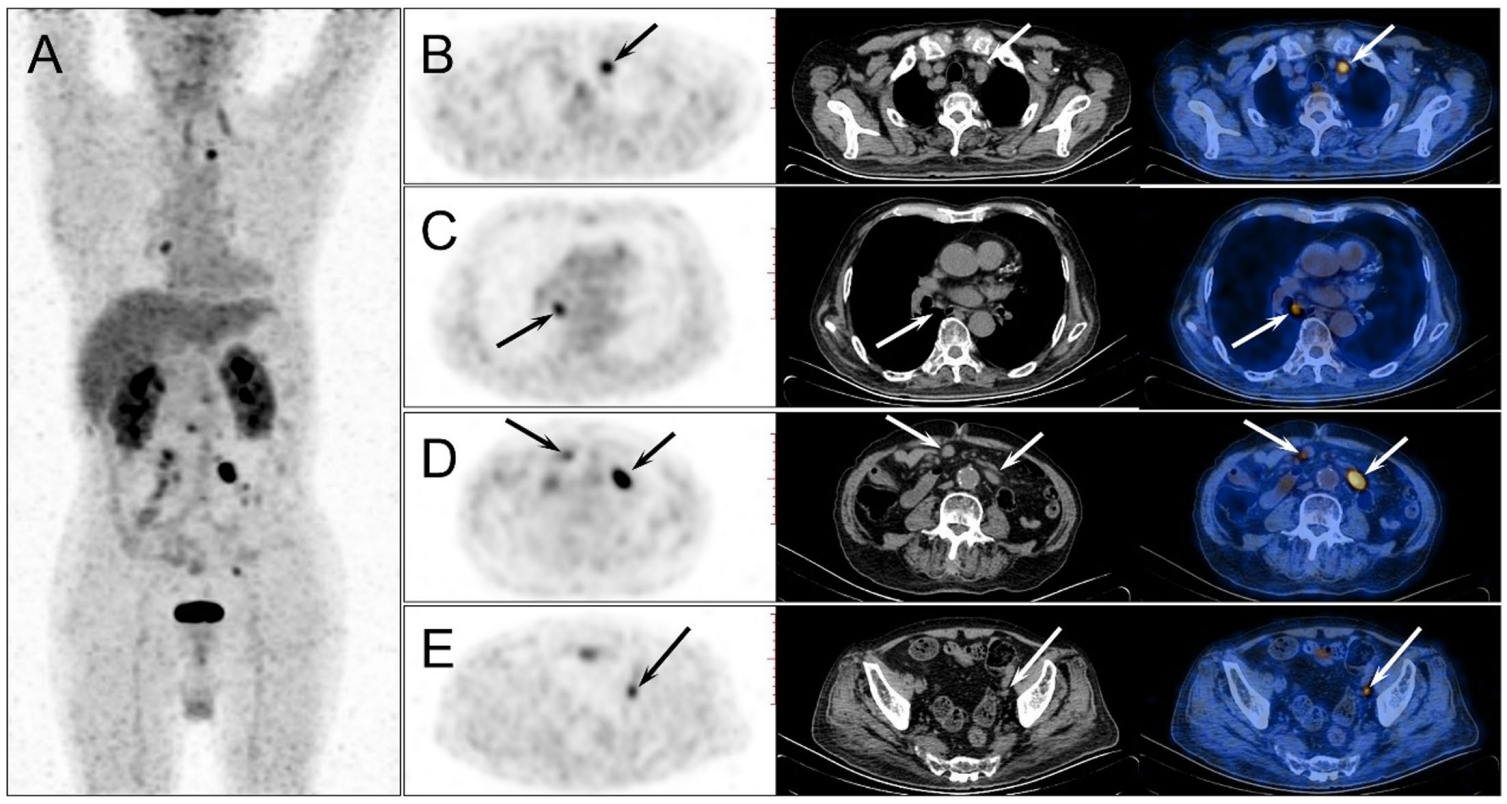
The second ^18^F-FDG PET/CT was performed 4 months after the HSCT. The MIP image (A) showed multiple new ^18^F-FDG-avid lesions in the chest and abdomen, while the previous hypermetabolic lesions all disappeared. On the axial images of PET, CT and PET/CT fusion, enlarged lymph nodes were observed in mediastinum (B,C), and abdominal and pelvic cavity (D,E) (arrows), which demonstrated intense ^18^F-FDG uptake with the highest SUVmax of 13.9 in the mesenteric lymph node.
